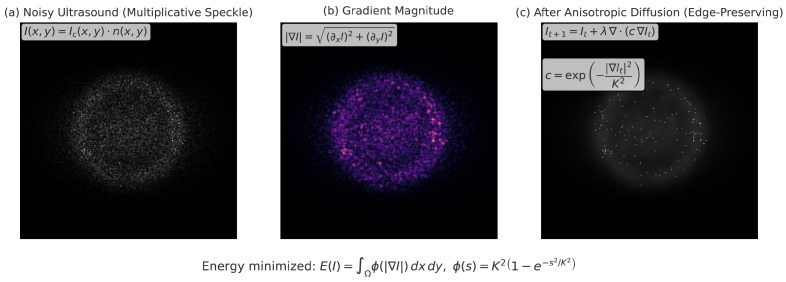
Anisotropic diffusion filtering applied to enhance structural continuity while removing speckle noise from fetal ultrasound images. This step ensures edge preservation for downstream segmentation and attention modules.
